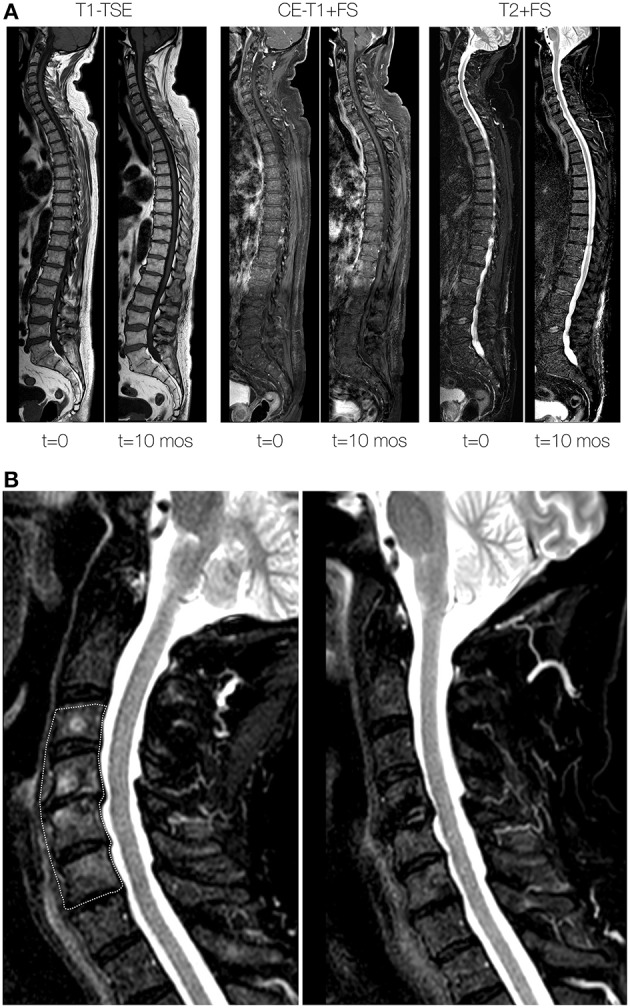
Vertebral lesions at baseline and during follow-up. (A) Total spine sagittal reconstructions of T1-weighted turbo spin-echo (TSE; left 2 panels), contrast-enhanced water-selective T1-weighted TSE (middle two panels) and T2-weighted sequences (right two panel) at baseline (t = 0) and 10 months (t = 10 mos) after initiation of treatment. At baseline, note the mottled appearance and lower signal intensity of the vertebral bodies on the T1-weighted sequences at baseline. At 10 months after treatment the signal intensity has returned to near normal signal intensity. (B) Zoomed detail of T2-weighted sequences at baseline (left) and 10 months after treatment (right) of the cervical spine. The area outlined by the dashed area at baseline signifies abnormally high signal intensity of the vertebral bone marrow, which returned to normal values at 10 months after treatment.
